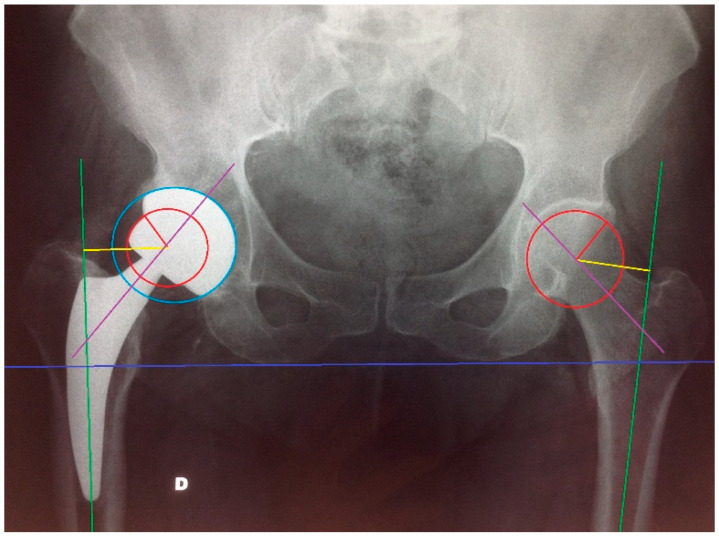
X-ray evaluation of joint geometry restoration.
